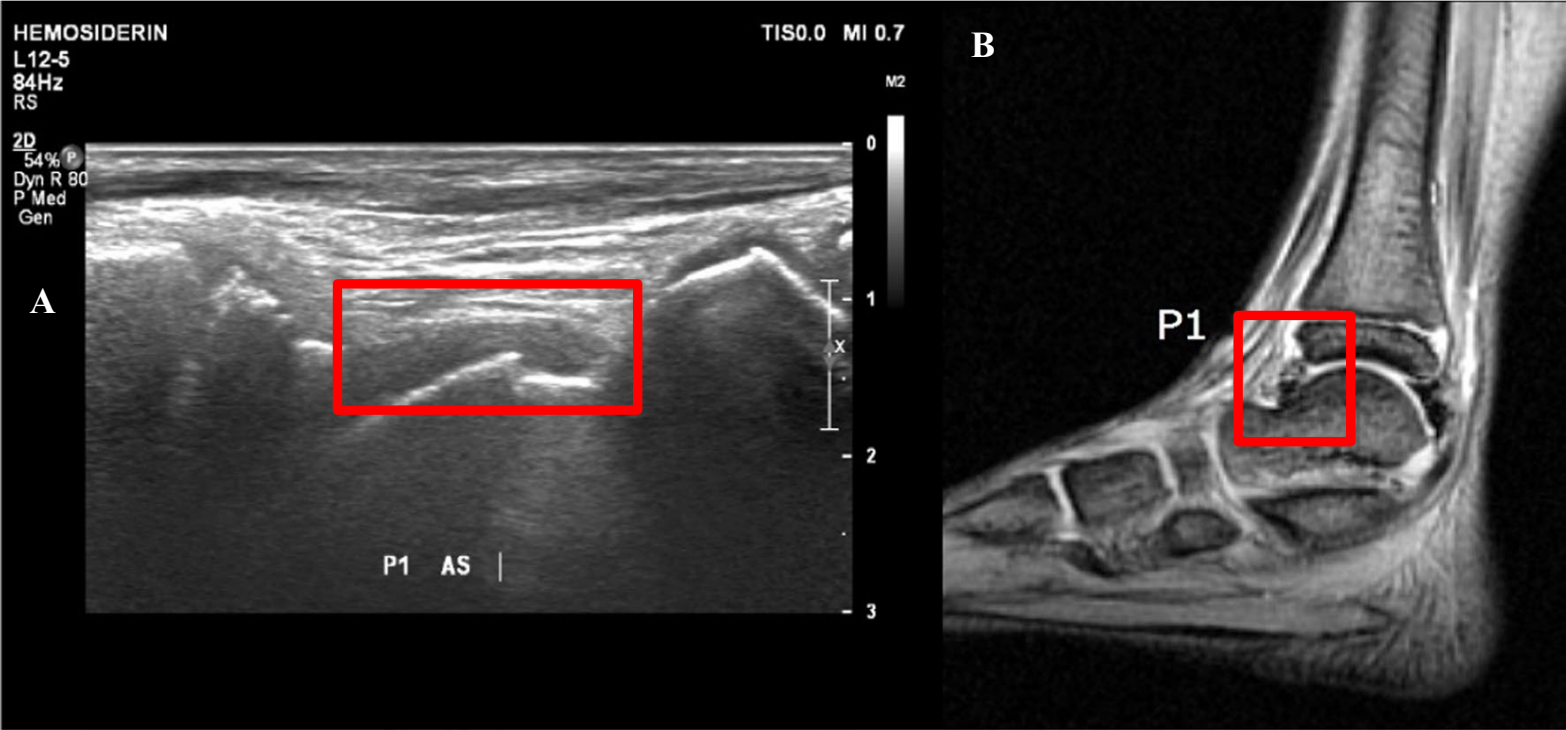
US examination at P1 location on the left ankle (a) showed synovial thickening as confirmed by MRI (b). Minimal hemosiderin (red box) is difficult to visualize on the US due to its closed proximity to the thickened synovium
